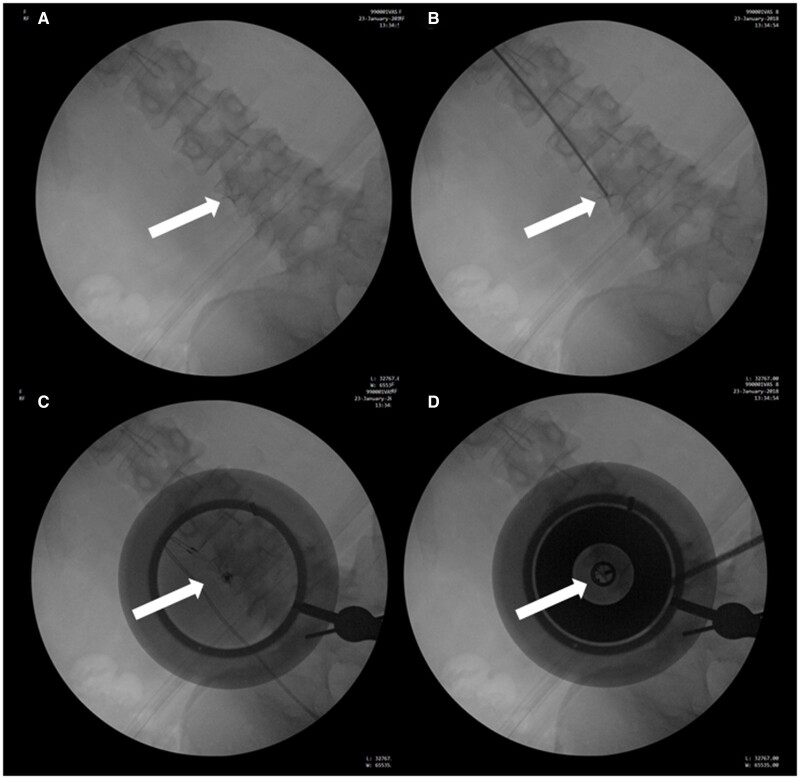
Targeting procedure. (A) X-ray image of the lumbar spine with an “X” marker (white arrow), attached to the C-arm image intensifier, positioned over the target area over the MB nerve along the lateral L4 pedicle. (B) X-ray image with an opaque rod denoting the skin site over the target area (white arrow), which is then marked with a permanent marker. (C) With the use of colinear lasers, the center of translucent mockup cradle is aligned to the mark on the skin, and the center of the “X” marker is aligned on the intensifier (white arrow). For accurate targeting, the laser spot position should be <6 mm from the center of the “X” from the image intensifier marker and <2 mm from the center of the skin marker. (D) Central mockup cradle is replaced with the more opaque Neurolyser Transducer, including an x-ray aimed at the center of the transducer (white arrow). The final targeting accuracy confirms the target in the center of the concentric circles.
